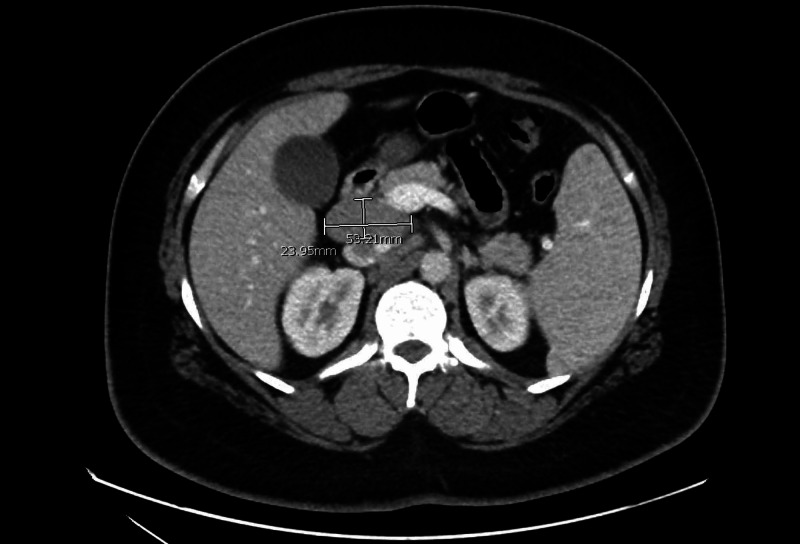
CT abdomen showing a transverse view of enlarged periportal lymph nodes measuring 2.4 cm × 5.3 cm in largest dimension (marked in white).
